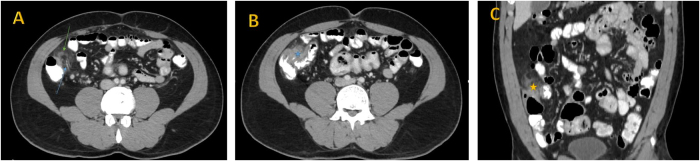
Selected axial (A + B) and coronal (C) abdomen CT scan with intravenous contrast images were done for our patient . They are showing a focal and oval shaped area of omental haziness is located along the anteromedial aspect of the ascending colon near the ileocecal valve (green arrow in A image and yellow star in C image), measuring 4.9 × 3.3 × 2.7 cm in craniocaudal, anteroposterior and transverse diameters respectively . Its associated with wall thickening of the adjacent part of ileocecal valve (blue star in B image). Note the small omental vessels entering the center of the haziness area (blue arrow in A image). These finding are suggestive of omental infarction.
